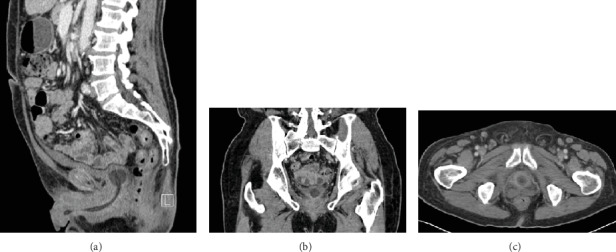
CT of the pelvis: (a) sagittal, (b) coronal, and (c) axial views of the prostate showing near resolution of the abscess with minimal residual fluid seen in the left posterior aspect at the base of the prostate.
